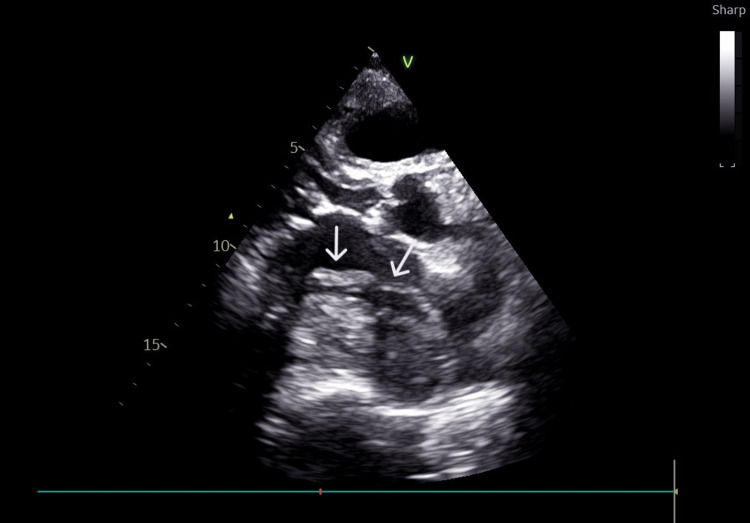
Echocardiographic image in the long-axis parasternal view showing a thrombus in the left atrium (indicated by the arrow)
